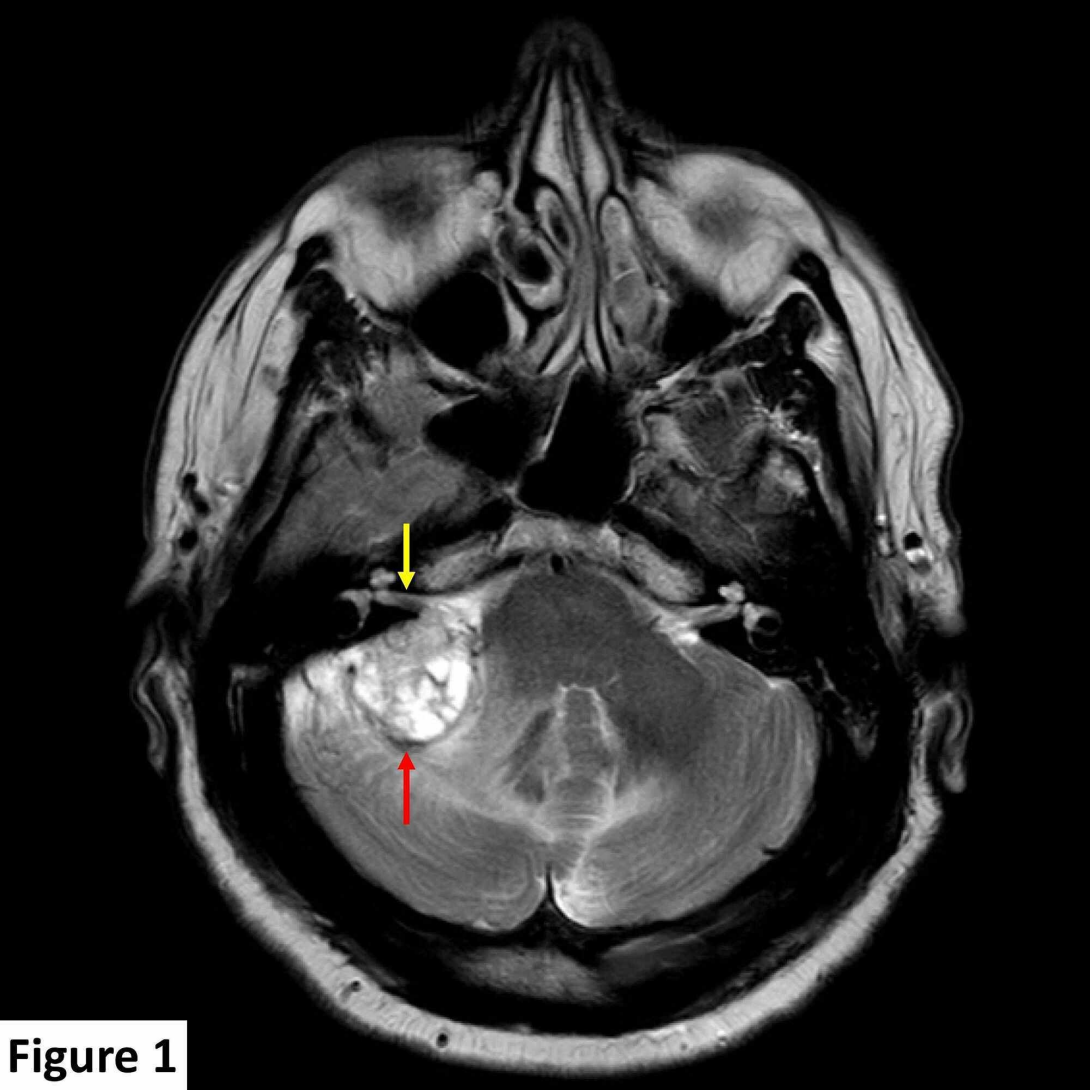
MRI scan at presentation. Axial T2 image showing a heterogenous T2 hyperintense lesion (red arrow) in the right cerebellopontine angle compressing the right middle cerebral peduncle, cerebellum, and fourth ventricle, with associated parenchymal high T2 signal change. The right internal auditory canal is spared (yellow arrow). MRI, magnetic resonance imaging
